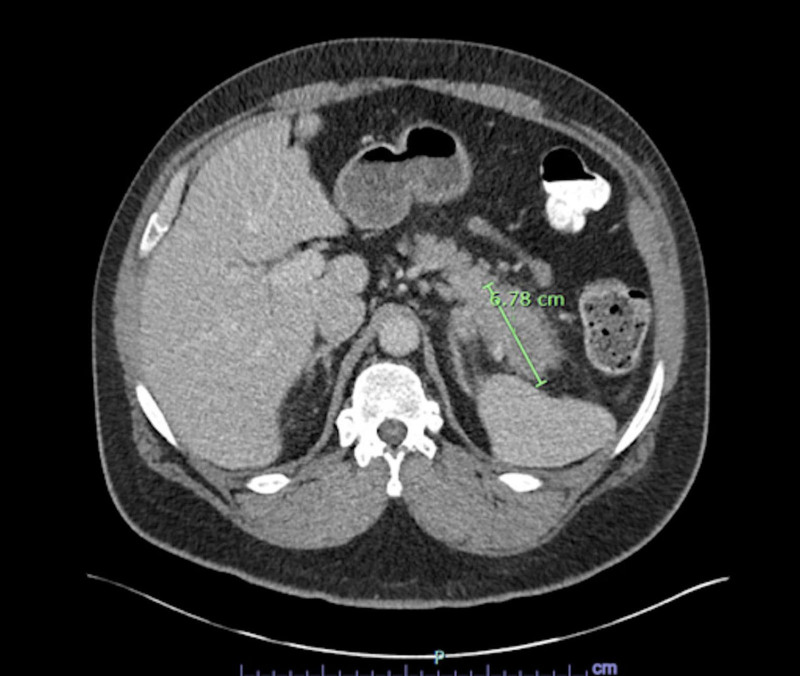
Computed tomography of the abdomen with contrast showing ill-defined mass-like thickening of the pancreatic tail.
